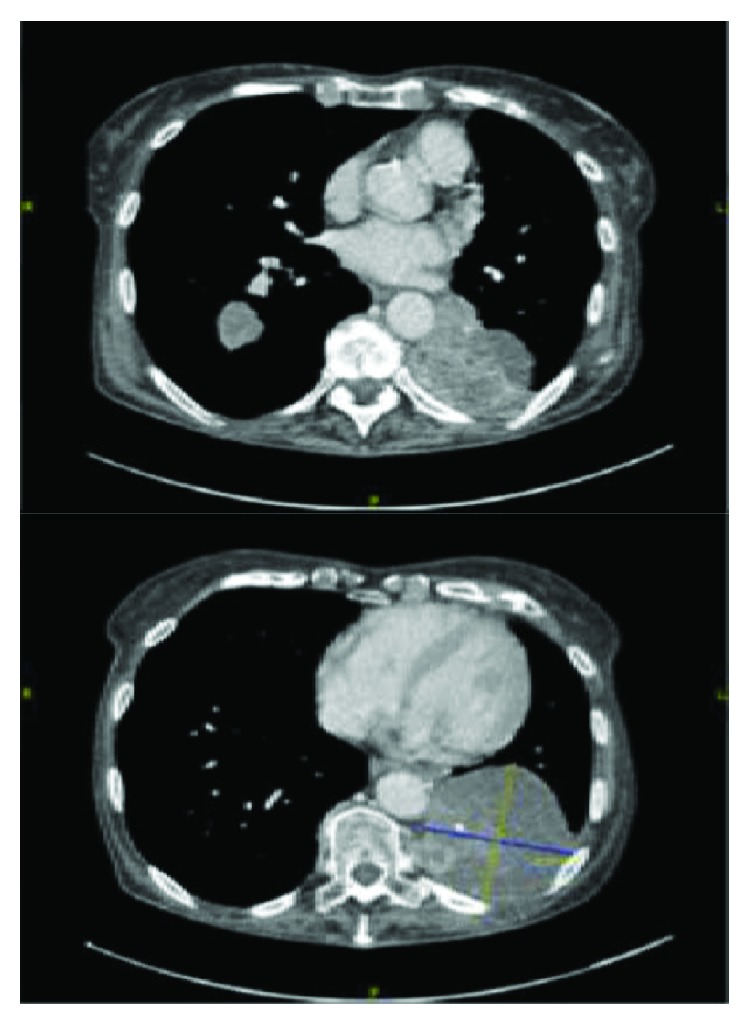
Restaging CT post 15 cycles of Nivolumab. Image on the top shows some reduction in RLL mass and the image on the bottom shows that the LLL mass had increased significantly.
